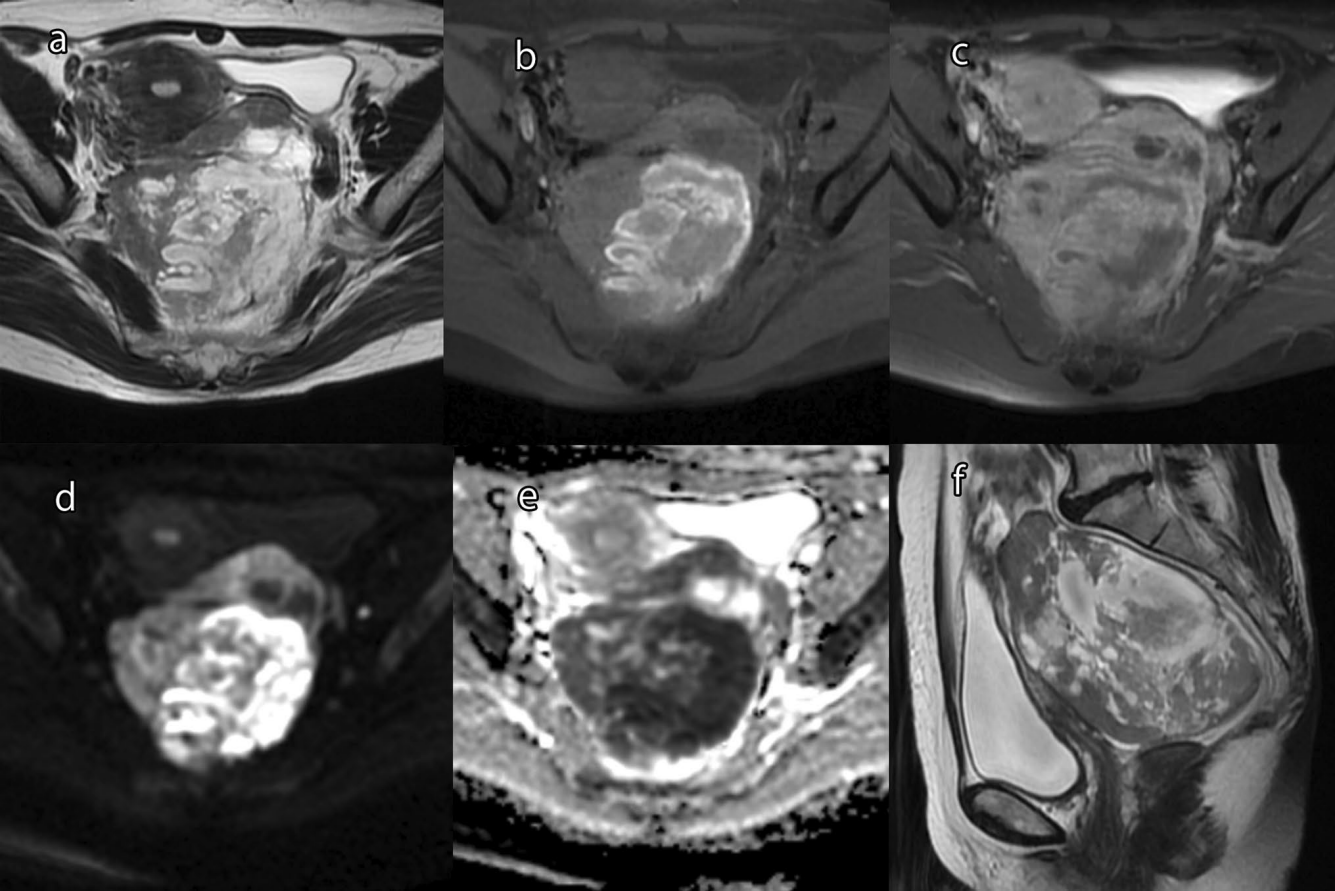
Endometrioid carcinoma in a woman in her 60s. a T2WI shows a mass in the left ovary consists of a cyst and solid components. b FS-T1WI shows a hyperintense area inside the mass, suggesting hemorrhage. c Gd-enhanced FS-T1WI shows that the solid components have the same degree of enhancement as the myometrium. d On DWI (high *b* value), the solid components are hyperintense compared with the myometrium. The hemorrhage area shows marked hyperintensity. e ADC maps show the solid components to be hypointense compared with the myometrium. f Sagittal T2WI shows the solid components to be multicentric
